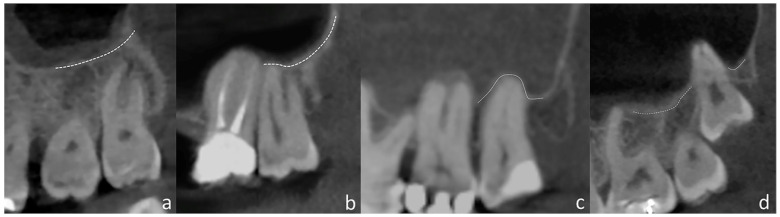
Relationship between roots and MS on CBCT: (a) apices distant from the MS floor; (b) MS floor touching the roots; (c) presence of alveolar domes; and (d) absence of bone covering.
